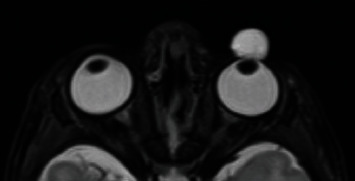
T2-weighted MRI of the orbits, axial view. Unilocular cyst arising from the anterior portion of the left globe.
